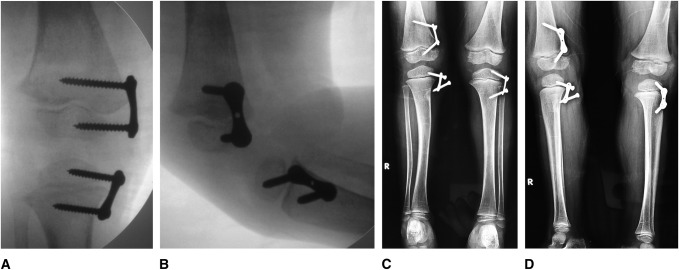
A–D, Radiographic appearance for the same patient as in Figure 3. A and B, Radiographs at time of implant insertion. C and D, Postoperative radiographic appearance (1.7 years). Despite massive implant disassembly especially of the tibial physis on the right limb with the severe genu valgum, full clinical correction was achieved.
